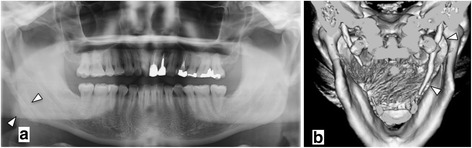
Panoramic radiographs (a) and three-dimensional computed tomography (b) revealed an elongated styloid process on the right side lateral to the ramus (*arrowheads*)
